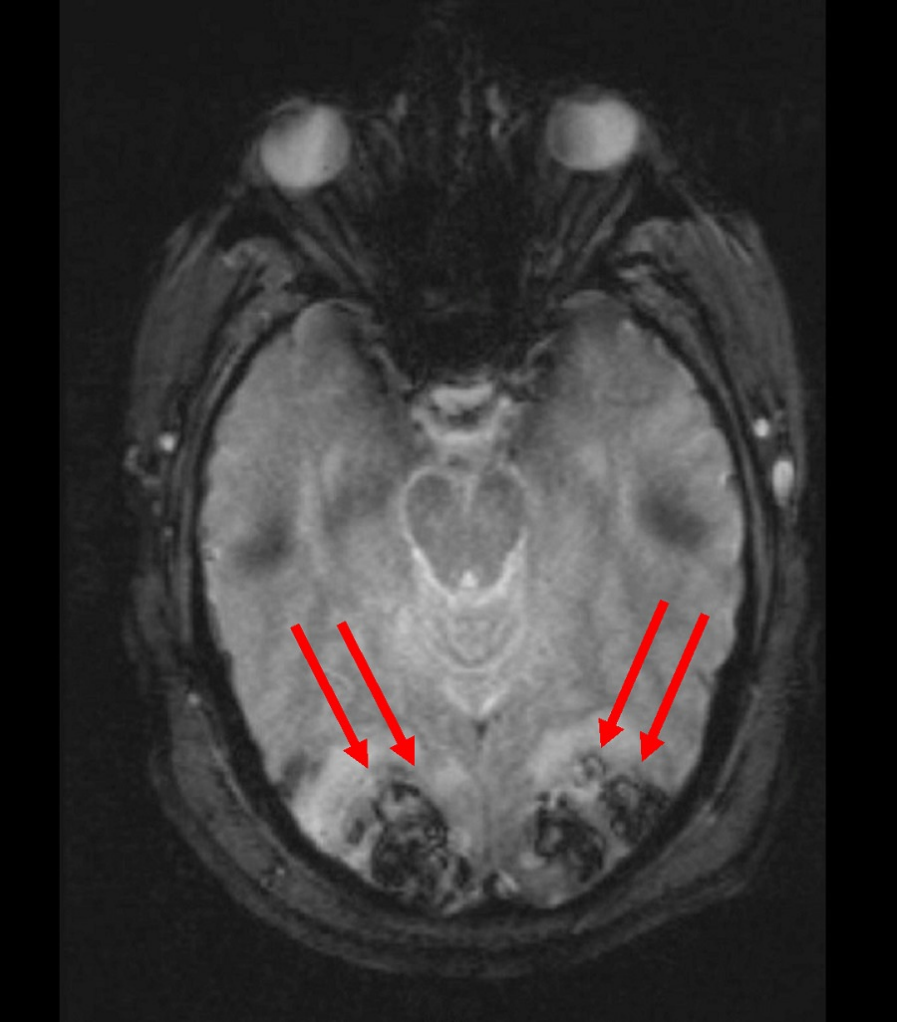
Magnetic resonance imaging of the head with gradient echo sequence showing bilateral occipital hemorrhages (two red arrows)
